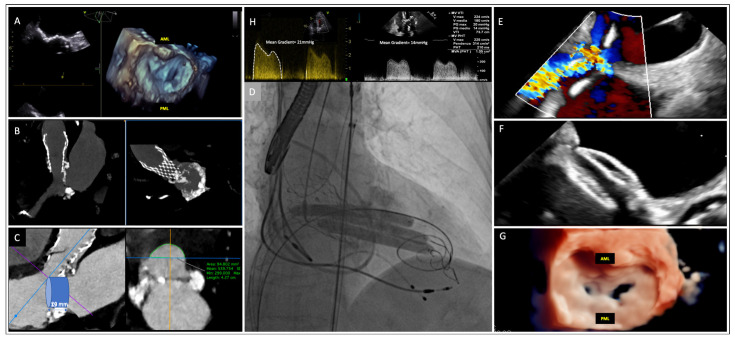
A case of transcatheter mitral valve lithotripsy without percutaneous balloon valvuloplasty for palliative treatment of an extremely calcified degenerative mitral valve stenosis. Panel A: TOE showing high calcification extending from the mitral annulus to both the anterior and posterior leaflets, causing severe mitral stenosis. Panels B,C: Preprocedural assessment CT scans showing the high risk of left ventricle outflow tract obstruction in the case of valve-in-MAC. Panel D: Simultaneous inflation of three lithotripsy balloons in the calcified mitral valve. Panels E,F: Closure of the iatrogenic interatrial septal defect with the Amplatzer ASD Septal Occluder 12 mm (Abbott). Panels G,H: Final TOE showing trans-mitral gradient reduction from 21 mmHg to 15 mmHg and an improvement in the mitral area from 0.4 to 0.8 cmq.
